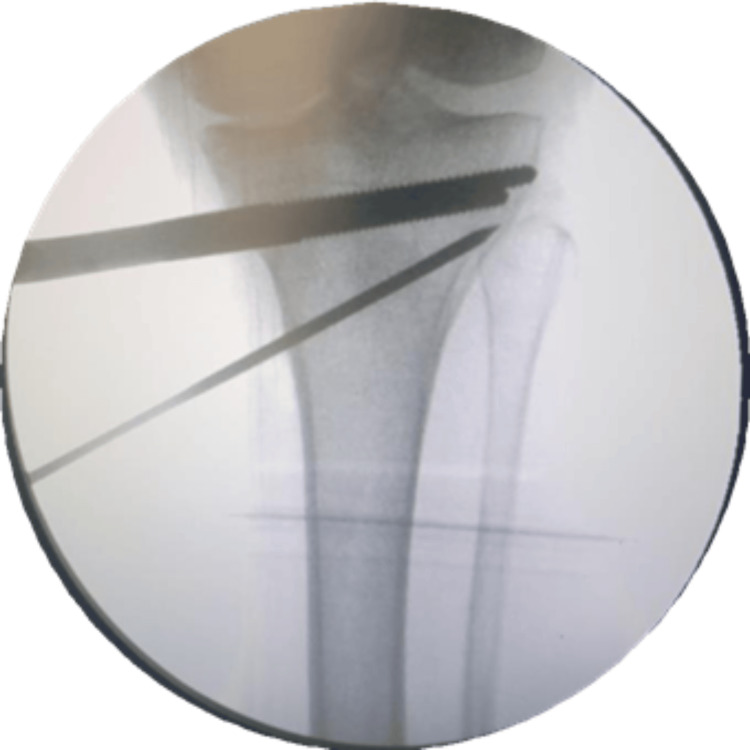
Intraoperative radiograph of two 6 mm tapered Schanz pins placed into proximal tibia parallel to each other and directing at the tip of the fibula.
